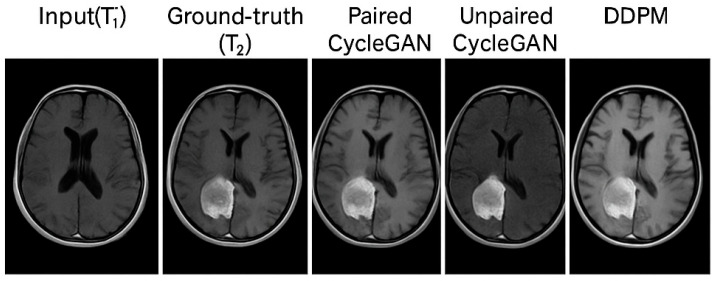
Qualitative comparison of MRI modality synthesis.
